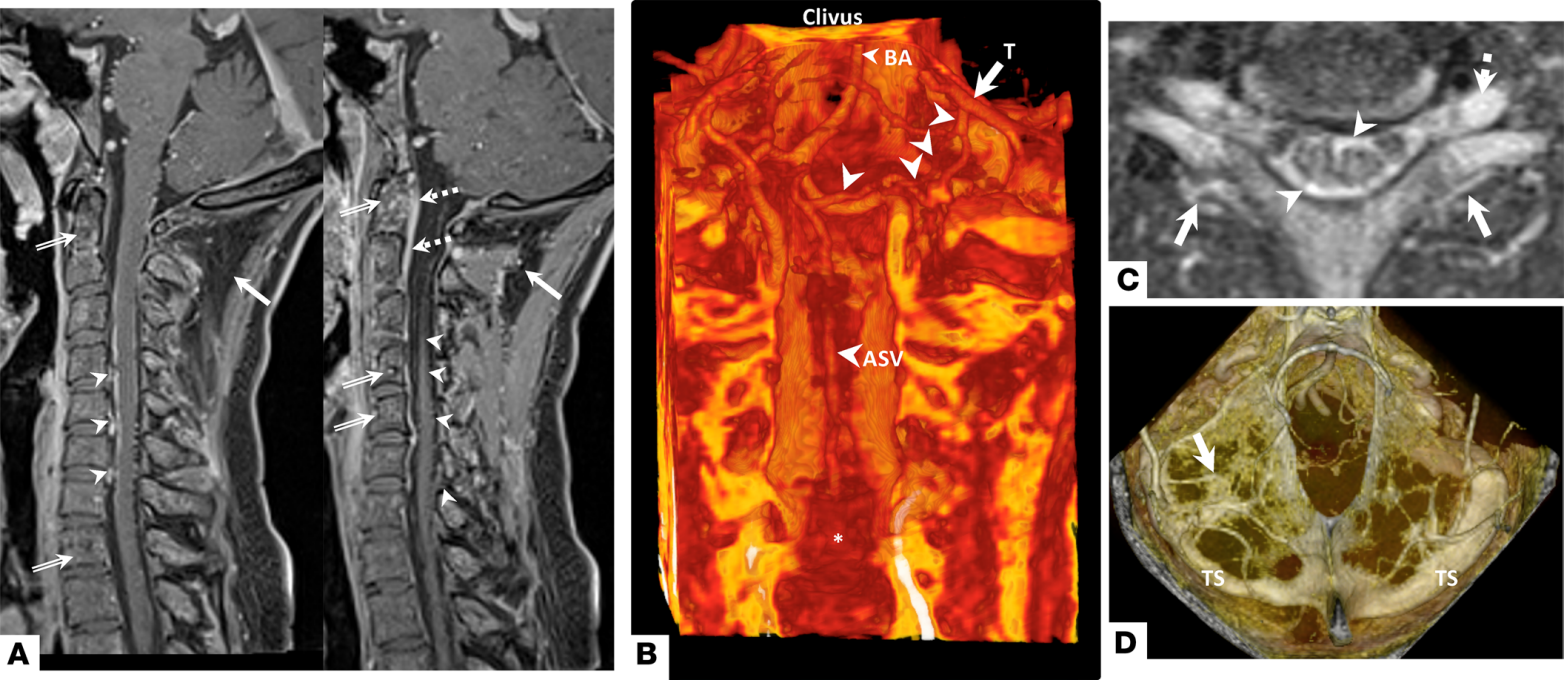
Meningeal and parenchymal venous malformations in HIF-2α gain-of-function syndrome patients. (A) Midsagittal T1-weighted MRI of the cervical spine with contrast of patient 1 shows the cavernous malformation of the neck (arrow) and multiple contrast enhancing vessels behind the C4–7 vertebral bodies (arrowheads). Contrast enhancement in abnormal appearing regions of vertebral bodies is also seen (double-lined arrows). Adjacent slice of the same MRI shows contrast enhancement of the meninges connecting to the veins of the malformation (arrowheads) on the left. Abnormal enhancement of the bone and ligaments (double-lined arrows) is found to be continuous with contrast enhancement within the dura posterior to the vertebral bodies (dashed arrows). The neck malformation is again seen (arrow). (B) Posterior coronal view of volumetric reconstruction of the same 3D T1-weighted postcontrast sequence MRI excluding parenchyma and highlighting bone and contrast shows a venous malformation (asterisk) originating from the anterior spinal vein (ASV) corresponding to the intradural contrast enhancing vessels in A; the ASV is connected (arrowheads) to the tentorium (T). BA, basilar artery. (C) Axial T1-weighted postcontrast MRI of the same patient shows the veins in the dural nerve root sleeve (dashed arrow) draining the veins of the spinal cord (arrowheads) with a widened anterior median sulcus; the enlarged external spinal column veins are also seen (arrows). (D) Volumetric reconstruction of T1-weighted postcontrast MRI of the head of patient 2 shows a developmental configuration of the tentorial veins and sinuses on the left (arrow); the left transverse sinus (TS) is smaller than the right.
